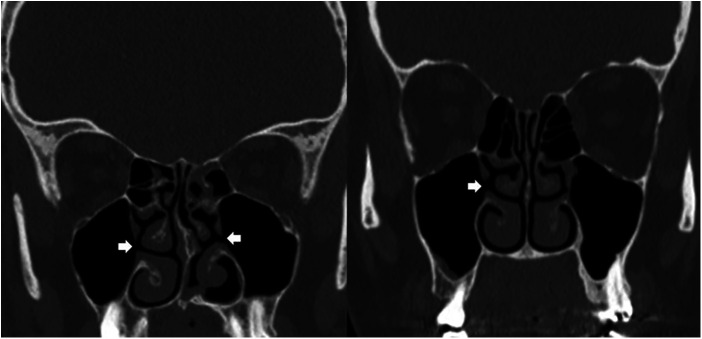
Coronal non-contrast CT images of the paranasal sinuses demonstrating accessory maxillary ostia (white arrows).
